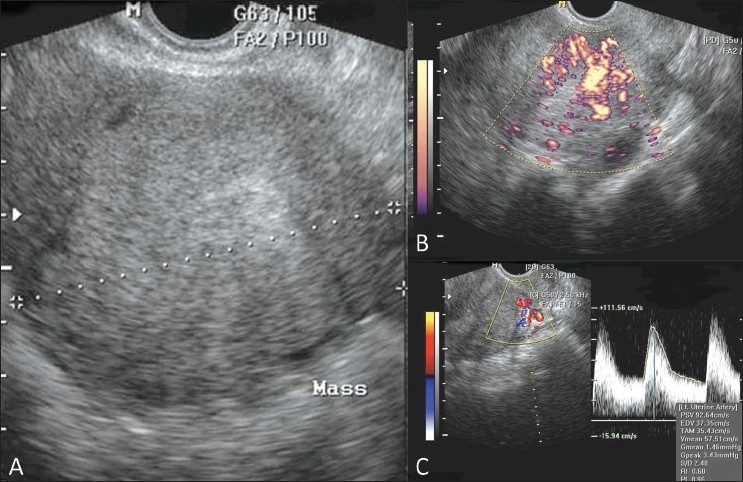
Adenomyoma. Transvaginal 2D gray-scale (A), power Doppler (B) and triplex Doppler (C) USG images show a uterine adenomyoma
